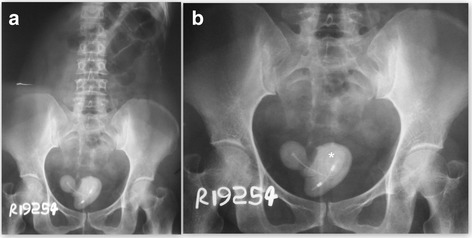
Plain radiographs. a The imprint of the intrauterine contraceptive device is seen in the center of the stone. b A magnified view of the X-ray showing the characteristic laminated appearance of the stone due to the concentric layers of stone material deposited around the intrauterine contraceptive device (*)
